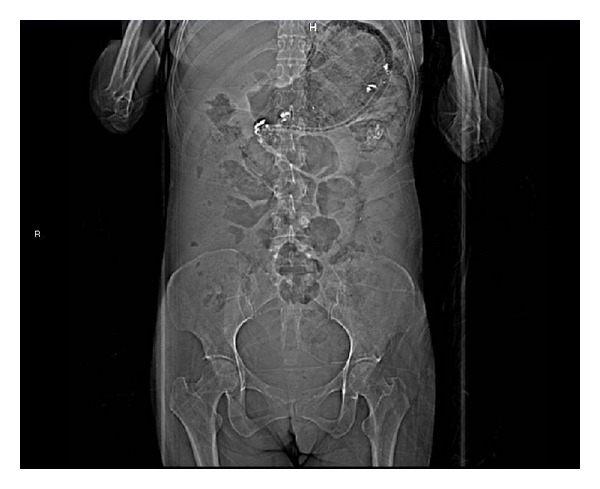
Abdominal scout image from a computed tomography.
